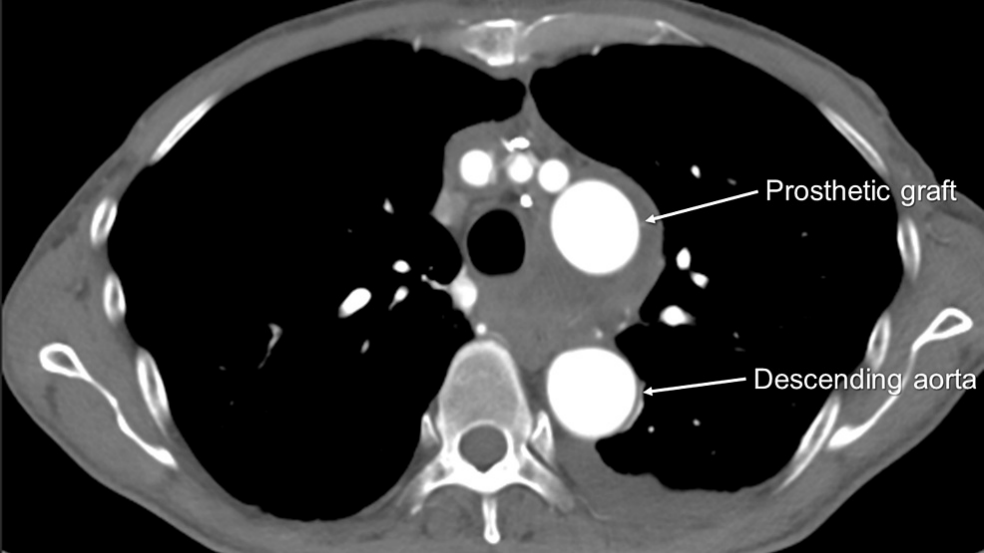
Postoperative contrast-enhanced computed tomography (CT) revealing a disappeared aneurysm
